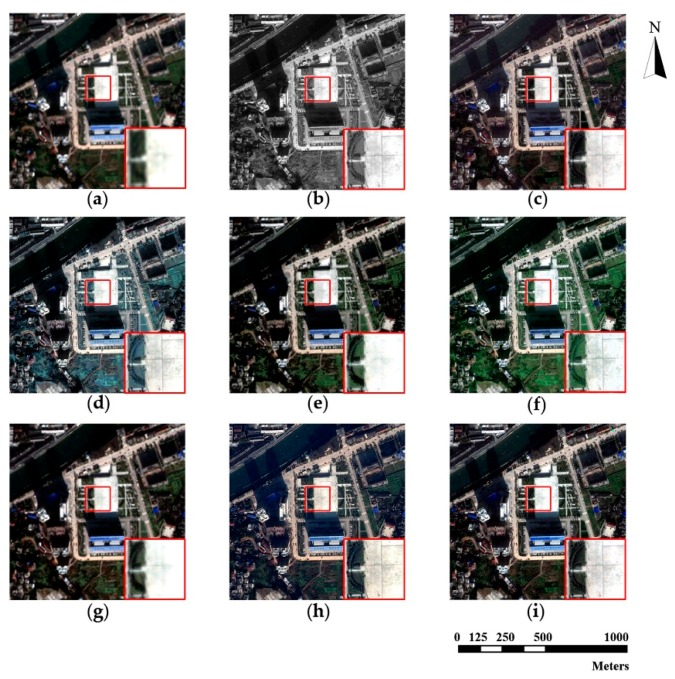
Pleiades original images and fusion results of different methods. (a) MS; (b) PAN; (c) GS; (d) AIHS; (e) WT-SR; (f) WT-IHS; (g) CT-ICA; (h) CSI; (i) proposed.
